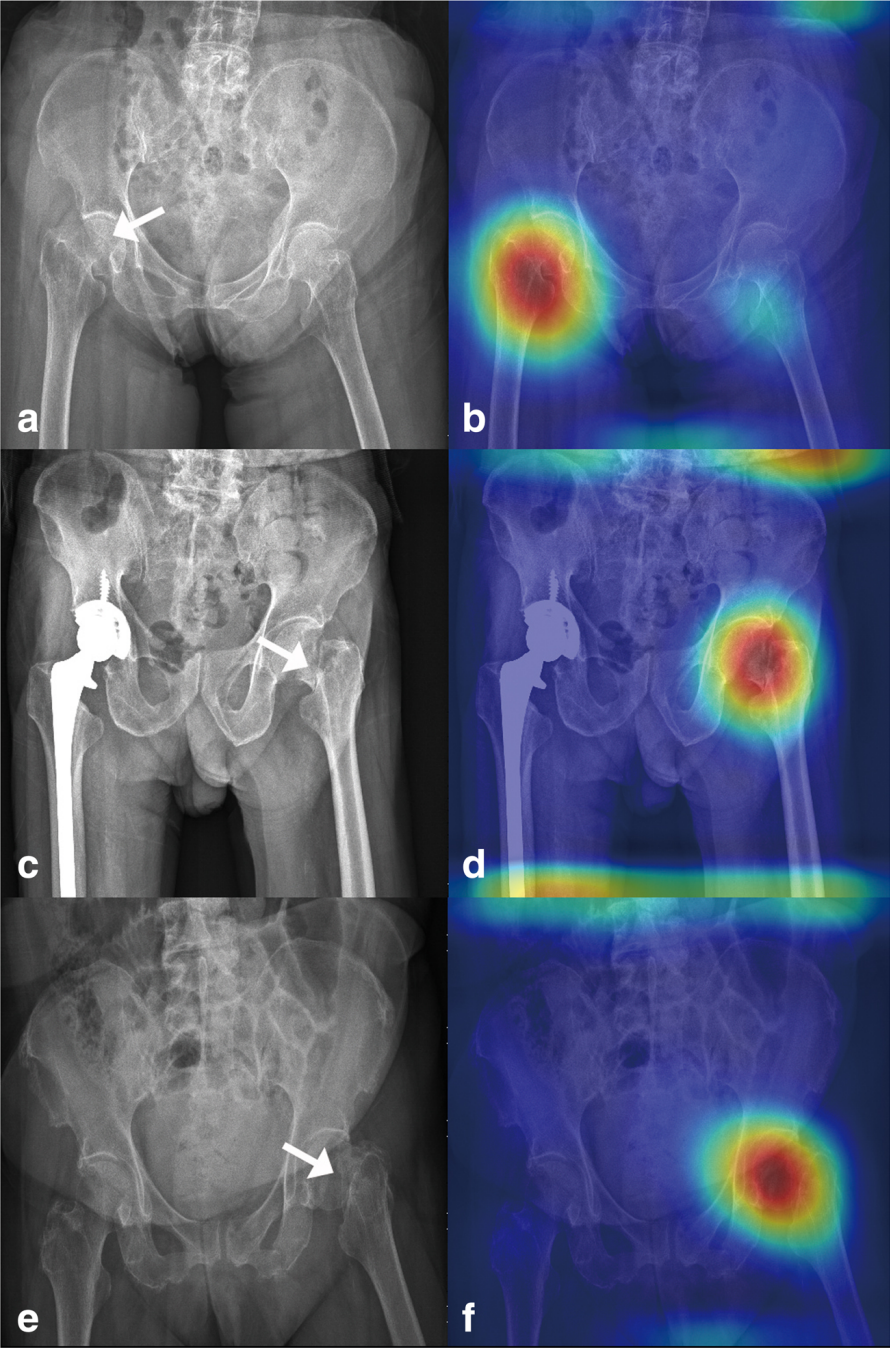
Grad-CAM-assisted image identification of hip fractures. a The original pelvic radiograph with a mildly displaced right femoral neck fracture (arrow) and b the image generated after applying the model with Grad-CAM, which visualizes the class-discriminative regions, as the fracture site. c PXR presenting a right total hip replacement with a left femoral neck fracture (arrow) and d a Grad-CAM-assisted image. e PXR with a mildly displaced left femoral neck fracture and f a Grad-CAM-assisted image
